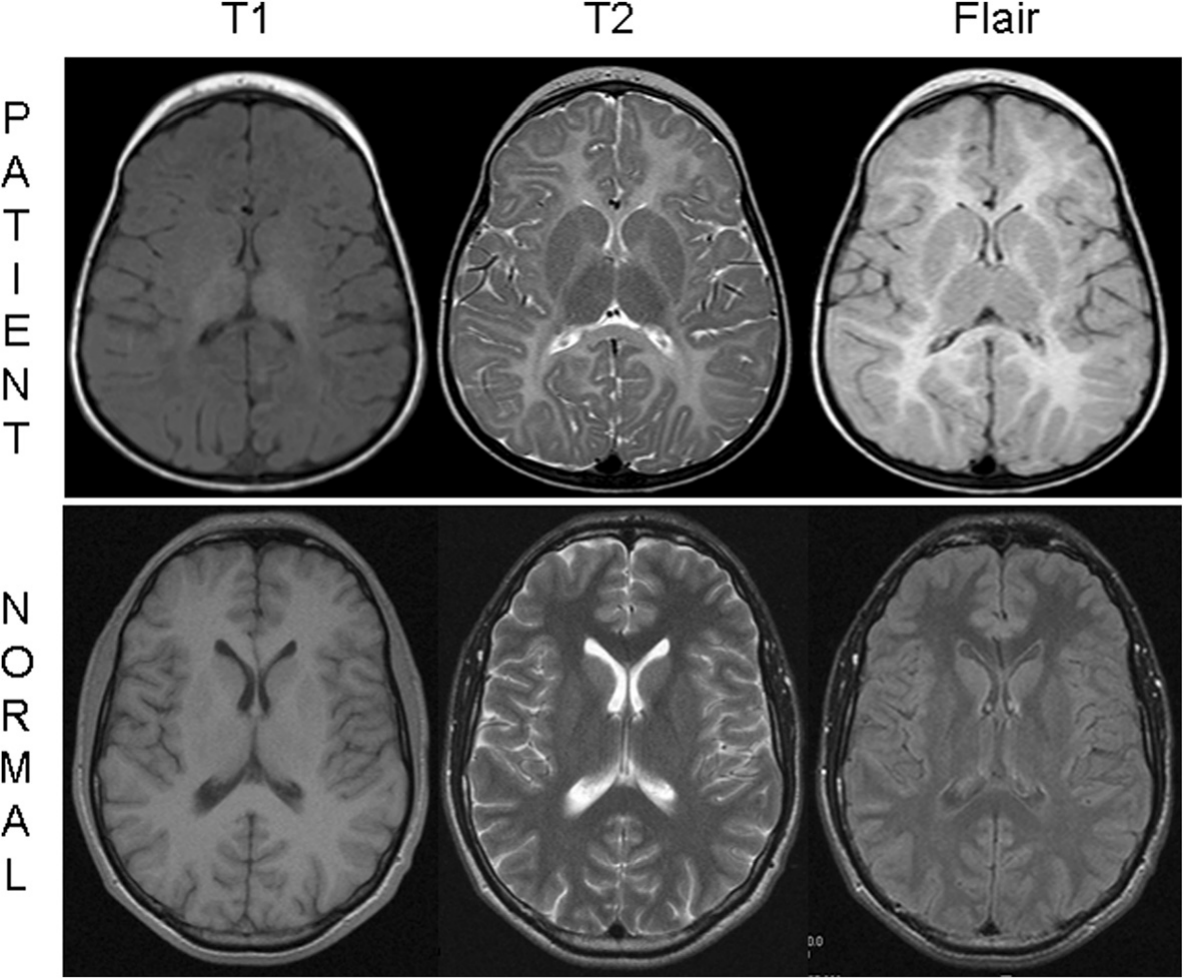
Patient’s brain magnetic resonance imaging (MRI). Sagittal T1-weighted MRI showed iso/hyposignal of the whole cerebral white matter, axial T2-weighted, and FLAIR images showing diffuse hypersignal of the hemispheric white matter, internal capsule, and corpus callosum in the patient at 2.5 years compared to an age-matched healthy child
